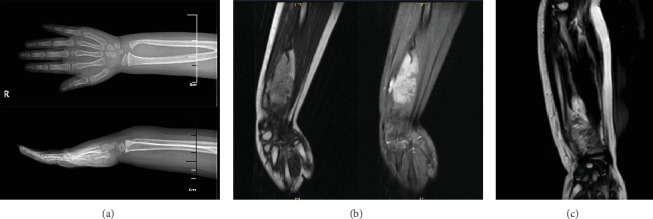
Radiologic examinations. (a) X-ray reveals abnormal bone quality of the right distal ulnar radius, characterized by roughness of the bone cortex and soft tissue swelling. (b) Magnetic resonance imaging revealed a T2 signal-enhancing mass in the right forearm with indistinct margins. (c) A magnetic resonance T2 image reveals tumor recurrence in muscle and the intermuscular space, accompanied by localized bone destruction.
